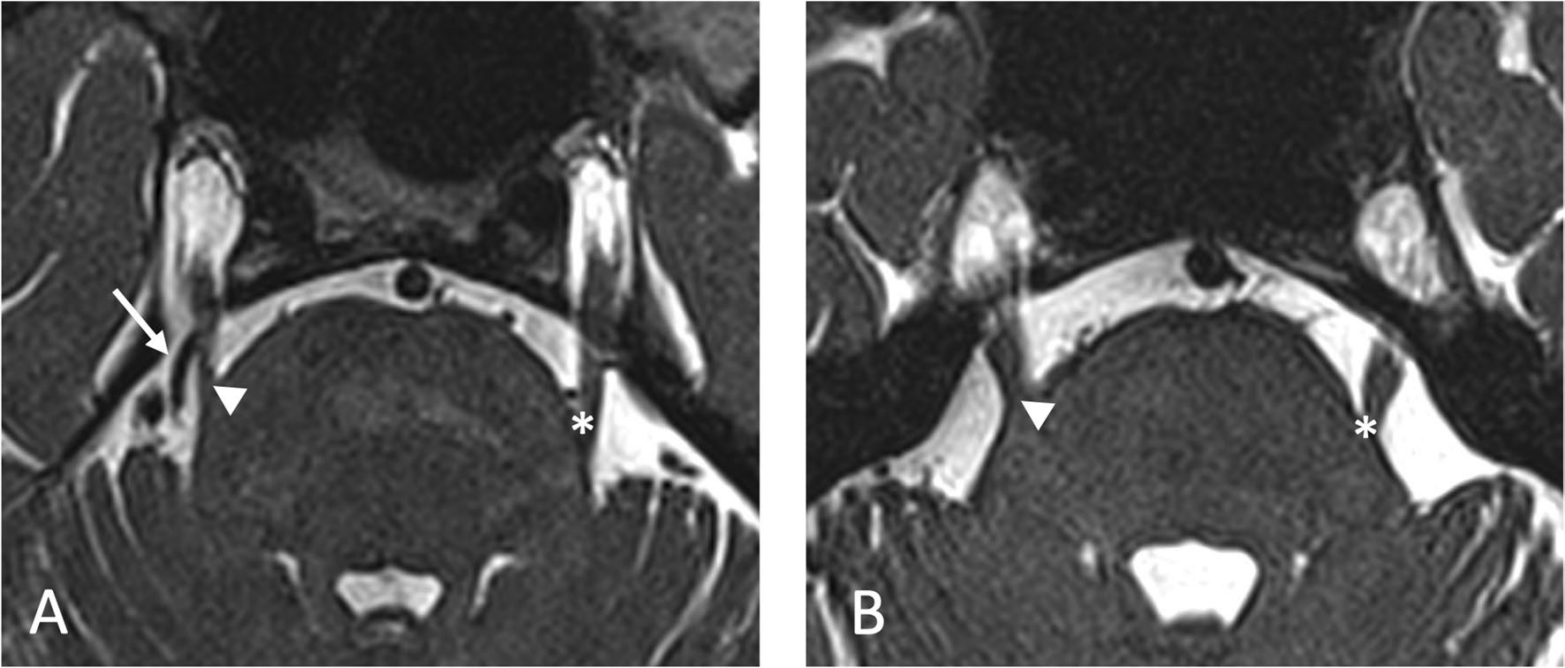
MRI CISS images of an exemplary patient with classical (A) and idiopathic (B) right TN. In (A) a vessel (arrow) impacts the right trigeminal root (arrowhead), which appears atrophic compared to the contralateral (star). In (B) no clear pathology affects the right trigeminal root (arrowhead), which appears similar to the contralateral (star)
